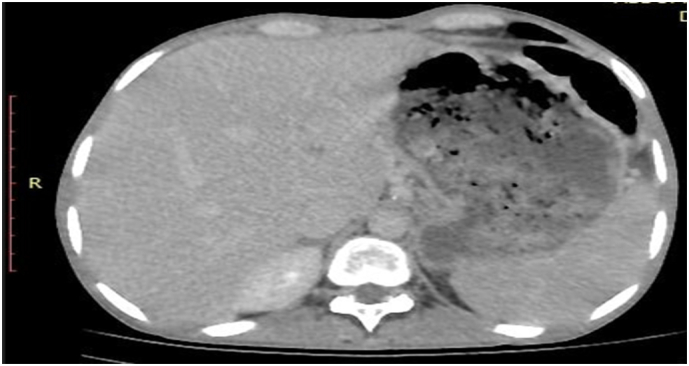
Abdominal CT scan in axil section which shows distended fluid filled stomach with outlet smooth tapering and multiple enlarged per pyloric lymph nodes.
